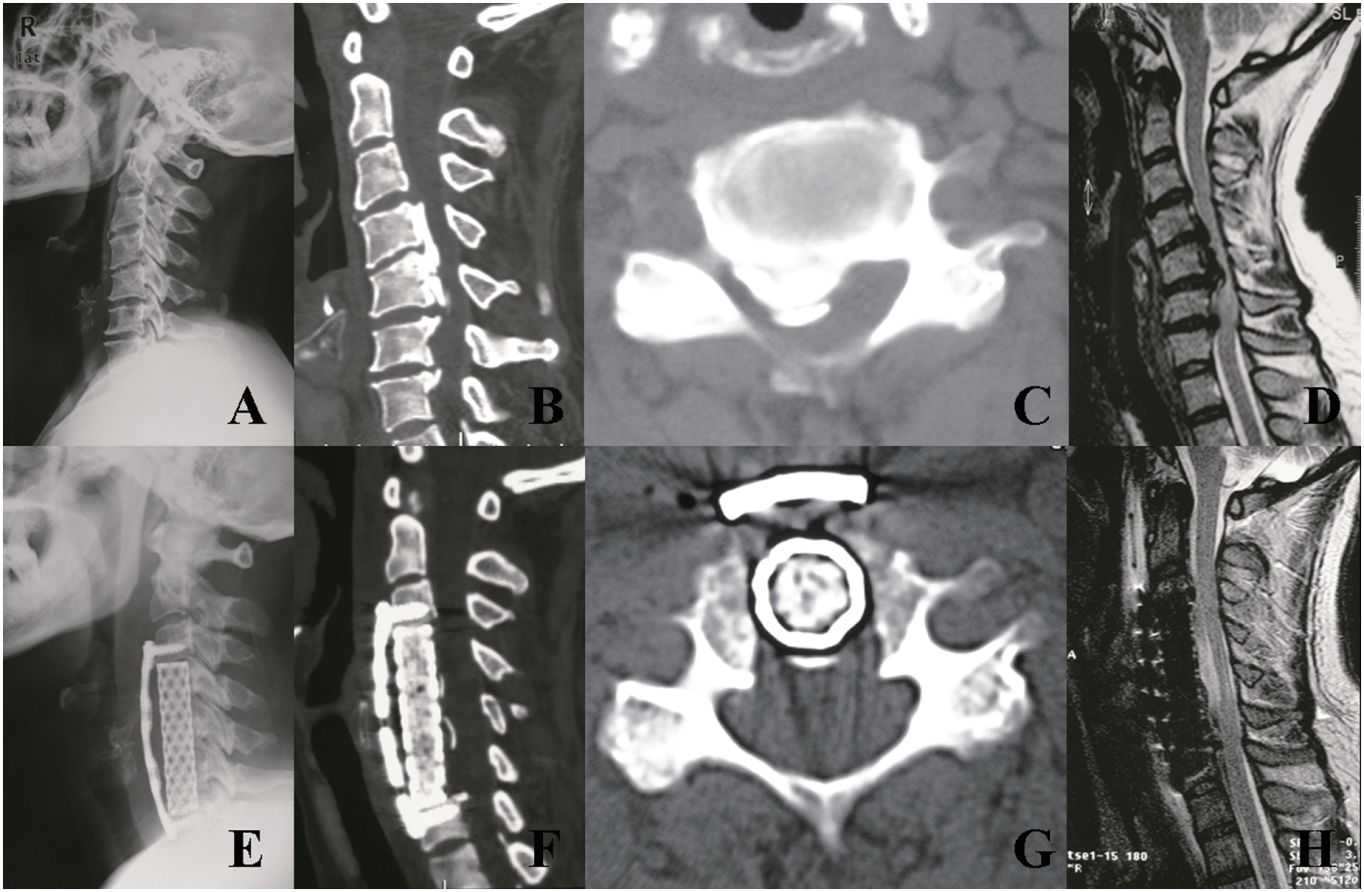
A 53-year-old men with a C4–C7 mixed-type OPLL associated with DO was treated by anterior cervical corpectomy and fusion. (A). Preoperative radiographic image at neutral position. (B, C). Preoperative sagittal and axial CT scans demonstrating a C4–C7 mixed-type OPLL with double-layer sign. (D). Preoperative MR image showed severe compression of the spinal cord. (E). Postoperative radiographic image showing C4–C6 corpectomy and fusion. (F, G). Postoperative sagittal and axial CT demonstrating complete resection of OPLL and floating of DO. (H). Postoperative MR image showed sufficient decompression of the spinal cord.
